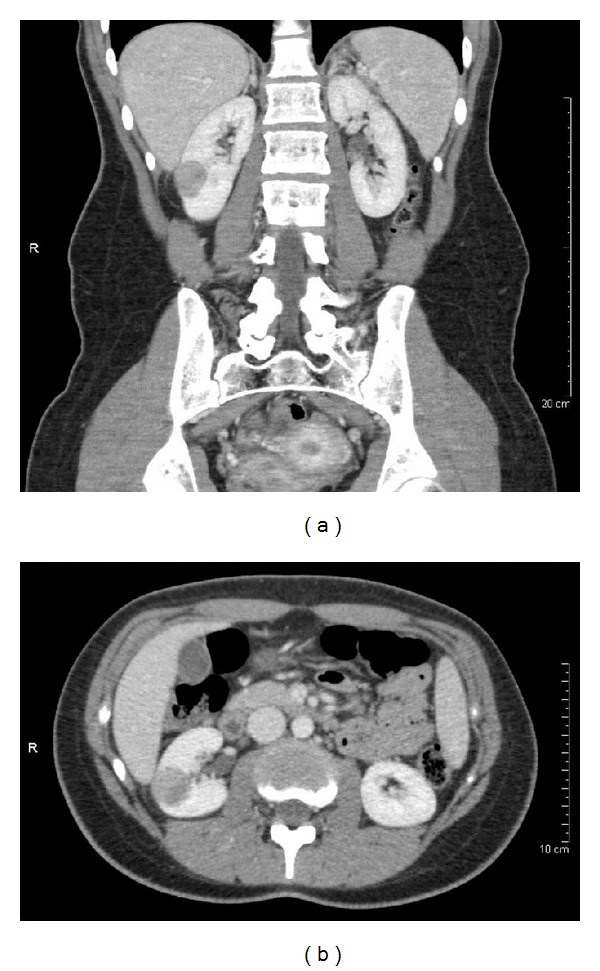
Imaging of TLFCK. (a) Coronal and (b) axial computed tomography with contrast showing a heterogeneous enhancing right renal mass.
